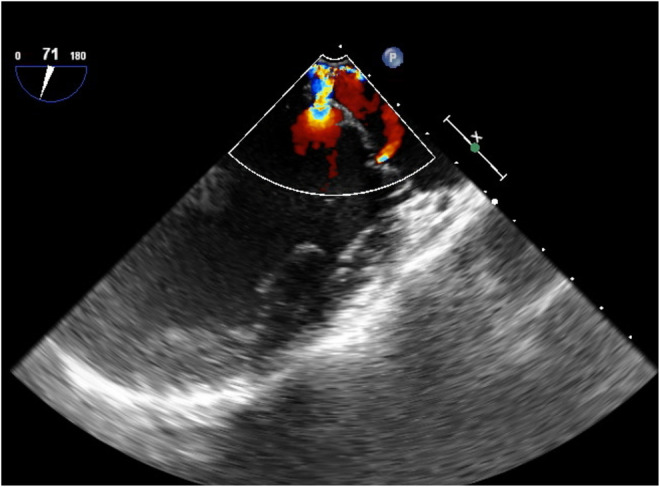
Representative images of transesophageal echocardiographic studies showing severe mitral valve regurgitation with ruptured mitral chordae tendineae.
